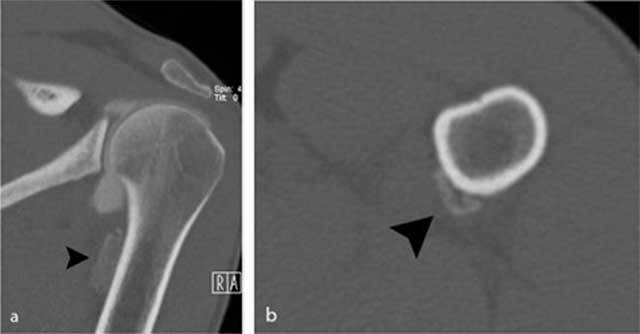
Additional CT images of the proximal humerus. a) Oblique coronal reformatted images and b) axial reformatted images reveal the presence of an extra-articular calcification (black arrowhead) near the insertion of the teres major tendon.
